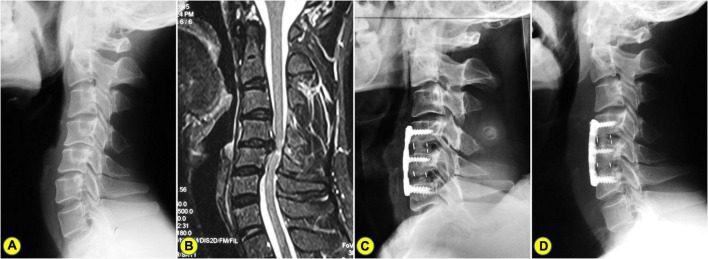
A 57-years-old man who underwent anterior cervical discectomy and fusion (ACDF). (A) The preoperative lateral X-ray shows the C2-7 Cobb angle and curvature index (CI) are 15^°^ and 9.49%, respectively. (B) Preoperative magnetic resonance imaging (MRI) showing C4-6 spinal cord compression combined with kyphosis. (C) A postoperative lateral X-ray showing ACDF. (D) On the lateral X-ray of 2.5 years after surgery, the C2-7 Cobb angle and CI were 7^°^ and 16.09%, respectively.
